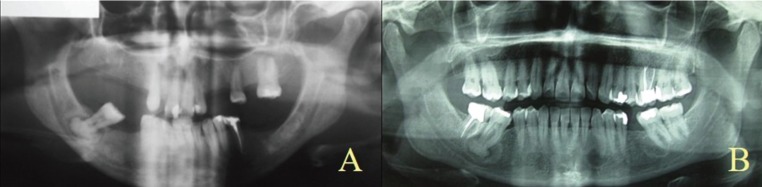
Orthopantomography. A) Case 1. Radio-opaque lesions in mandible localized on the periapex of tooth 4.7, the edentulous area corresponding to tooth 3.6 and 3.7 and periapical radiolucent lesion periapical of tooth 1.1. B) Case 2. Periapical radio-opaque lesion of tooth 4.7 and periapical radiolucent lesions in teeth 3.3 and 3.7.
